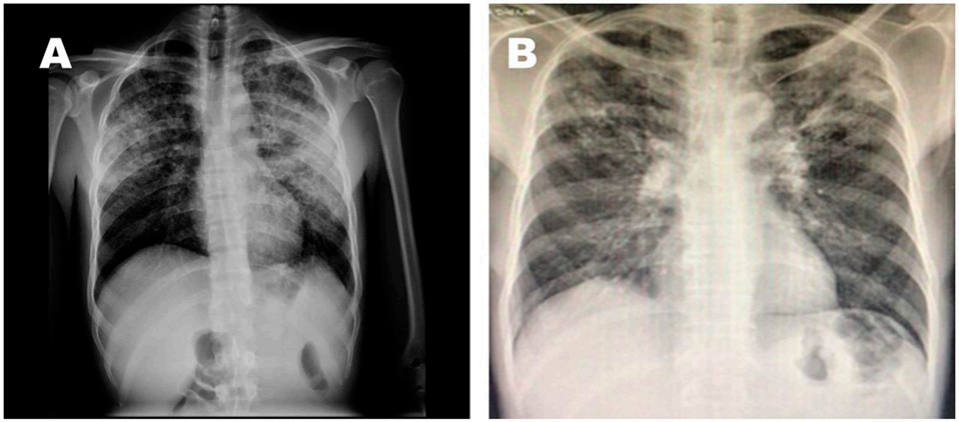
Thorax radiography: Patient (A) demonstrates bilateral interstitial infiltration. Patient (B) demonstrates left apical interstitial infiltration.
